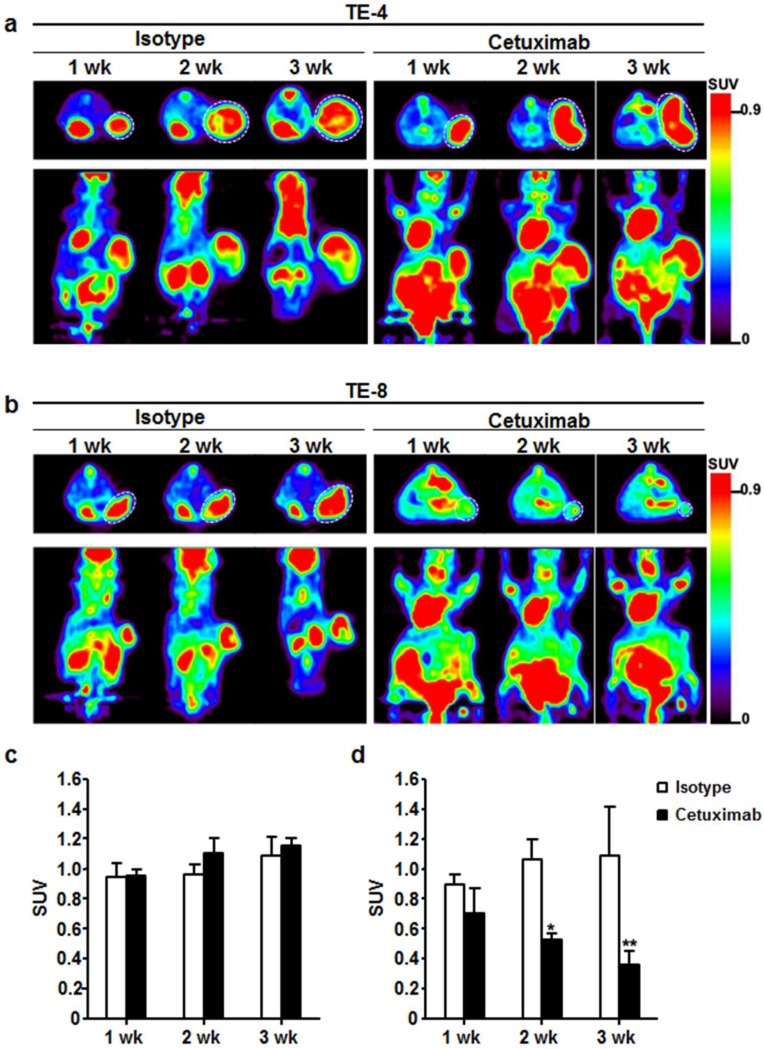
Therapeutic response monitoring with cetuximab treatment using ^18^F-FDG-PET imaging. (a) For 3 weeks, ^18^F-FDG uptake was maintained or slightly increased in TE-4 tumor regardless of treatment. (b) ^18^F-FDG uptake gradually decreased for 3 weeks in TE-8 tumors by cetuximab treatment. On the other hand, ^18^F-FDG accumulation gradually increased with isotype treatment in TE-8 tumors. (c) and (d) Quantitative indices of ^18^F-FDG uptake in TE-4 and TE-8 tumor represented as SUV. * *p* < 0.05;  *p* < 0.01. White dotted circle is tumor.
